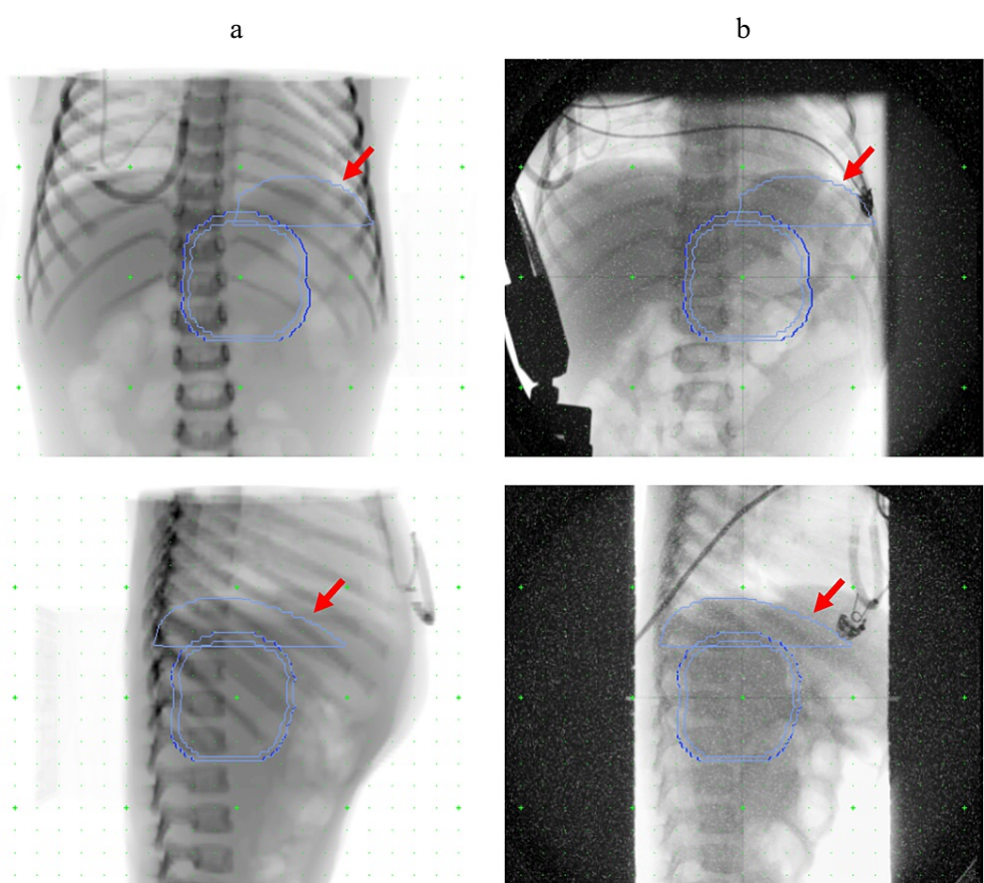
Frontal (upper) and lateral (lower) views during patient positioning (a) Digitally reconstructed radiographs obtained from the XiO-M treatment planning system (RTP). (b) X-ray images during patient setup on vertebral body matching. Red arrows indicate left diaphragm contours delineated on XiO-M RTP. Other contours in each image show the clinical target volume and planning target volume.
